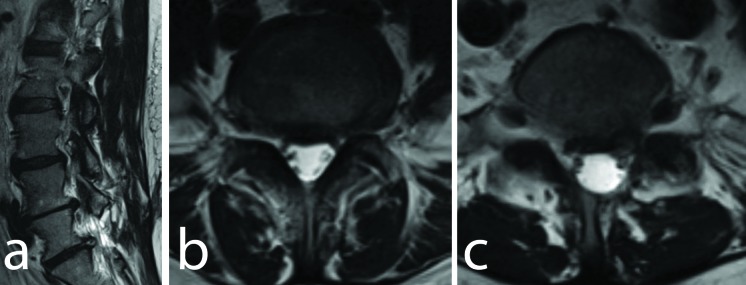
Axial and Sagittal T2W Images (a) Preoperative T2-weighted sagittal magnetic resonance imaging (MRI) scan demonstrating the presence of left L4-5 and L5-S1 disc herniations. (b) Axial T2-weighted MRI at L4-5 level. (c) Axial T2-weighted MRI at L5-S1 level demonstrating foraminal compression but with no evidence of an arachnoid cyst.
